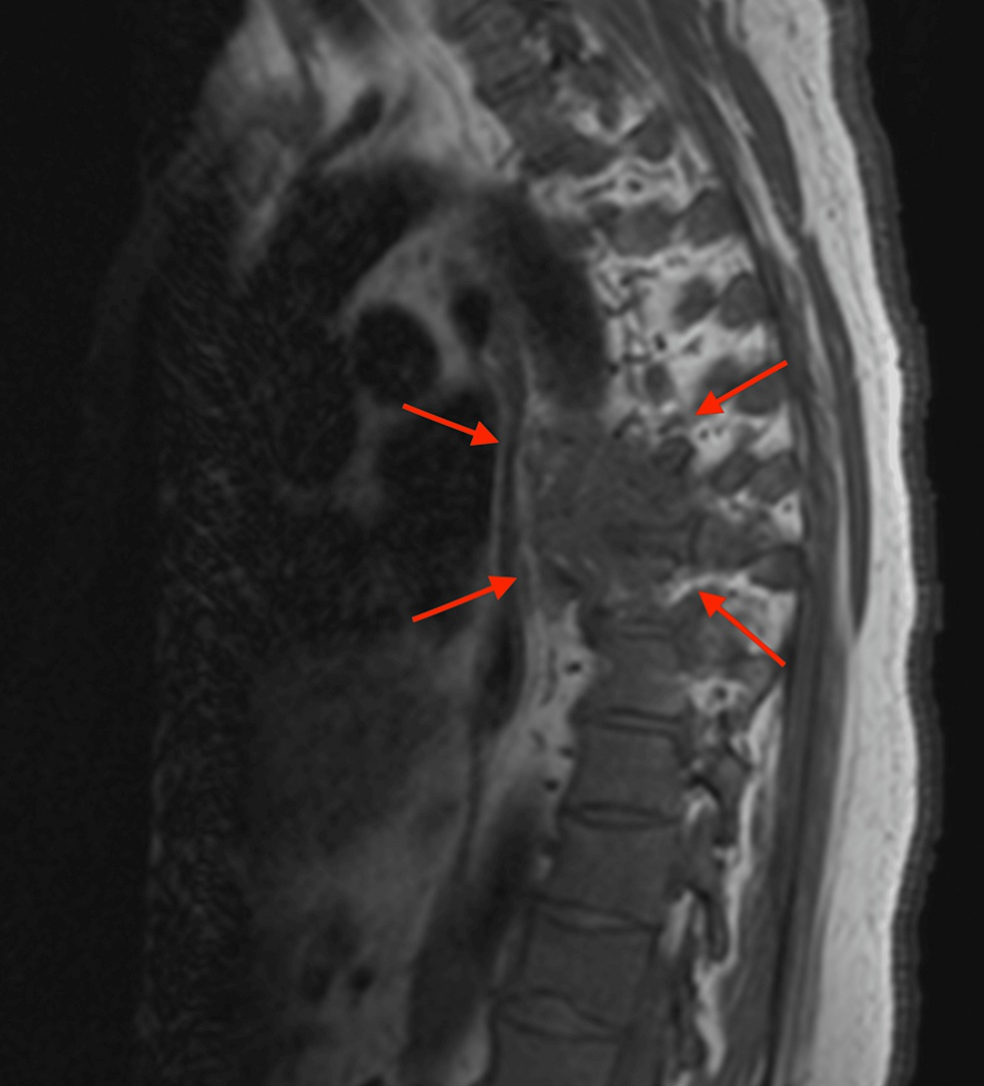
T2-weighted sagittal MRI with IV contrast showing widespread osseous metastatic neoplasm, with significant bone destruction involving the mid-thoracic spine, centered around T7
